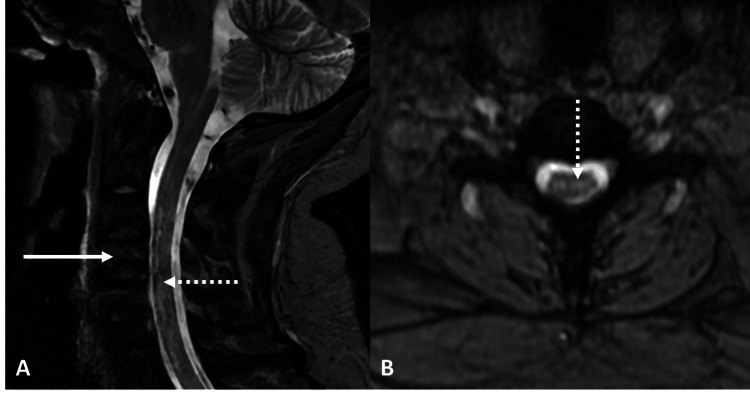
MRI of the cervical spine A & B: Geographical areas of T1 hypo and T2-weighted short tau inversion recovery (T2/STIR) hyperintensity (solid white arrow) noted involving the body of C2-C6 vertebrae. No evidence of involvement of posterior elements. Patchy areas of T2/ STIR hyperintensity (dotted white arrow) are noted in the cervical spinal cord adjacent to it.
